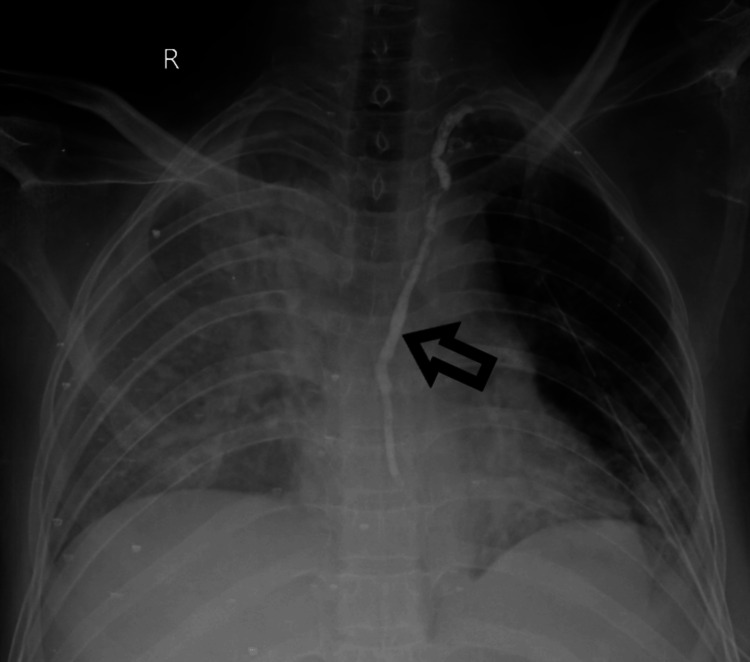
Post-embolization chest X-ray PA view showing hyperdense material in the main thoracic duct (arrow), suggesting complete embolization with NBCA glue and the absence of pleural effusion. PA: Posteroanterior; NBCA: N-Butyl Cyanoacrylate.
